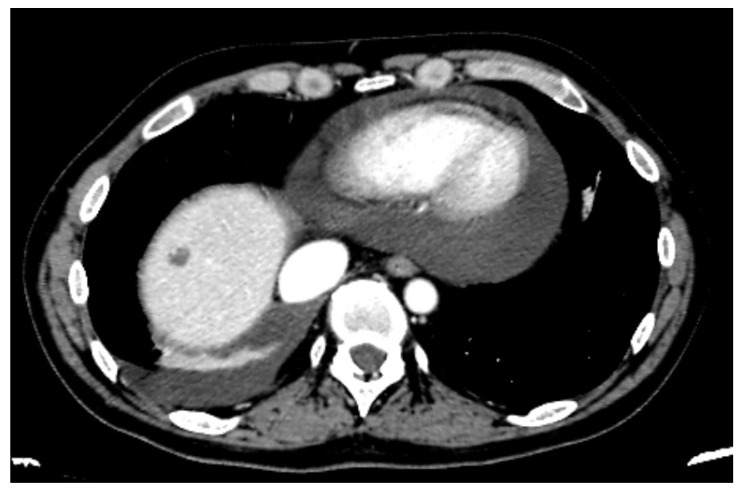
Preoperative CT scan revealing pericardial and right pleural effusion.
